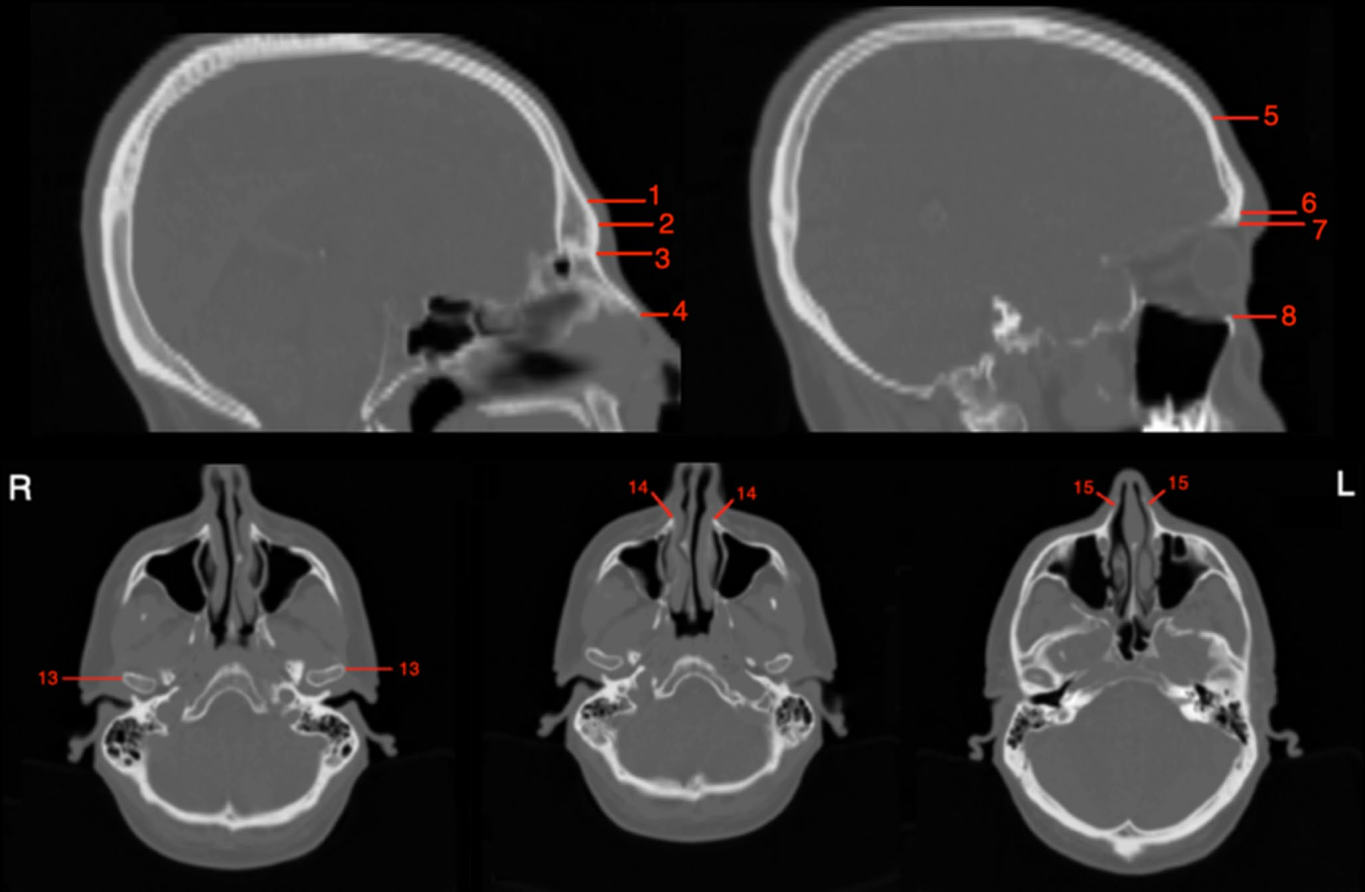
While the sagittal planes of the CT slices (upper row) were used to measure the midline and some of the bilateral cranial landmarks for the 2D measurement method, the transverse planes (lower row) were used to measure the rest of the bilateral cranial landmarks
